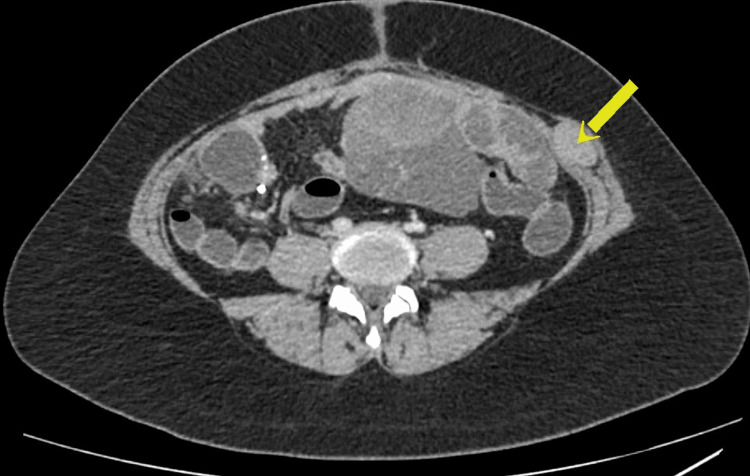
Craniocaudal view of the postoperative CECT with lateral abdominal wall metastases (yellow arrow). Arrow pointing at one of the suspected abdominal wall metastatic lesions. Lesion is positioned on the left lateral abdominal wall, and it exhibits a similar density to the surrounding soft tissue. CECT: contrast-enhanced computed tomography
